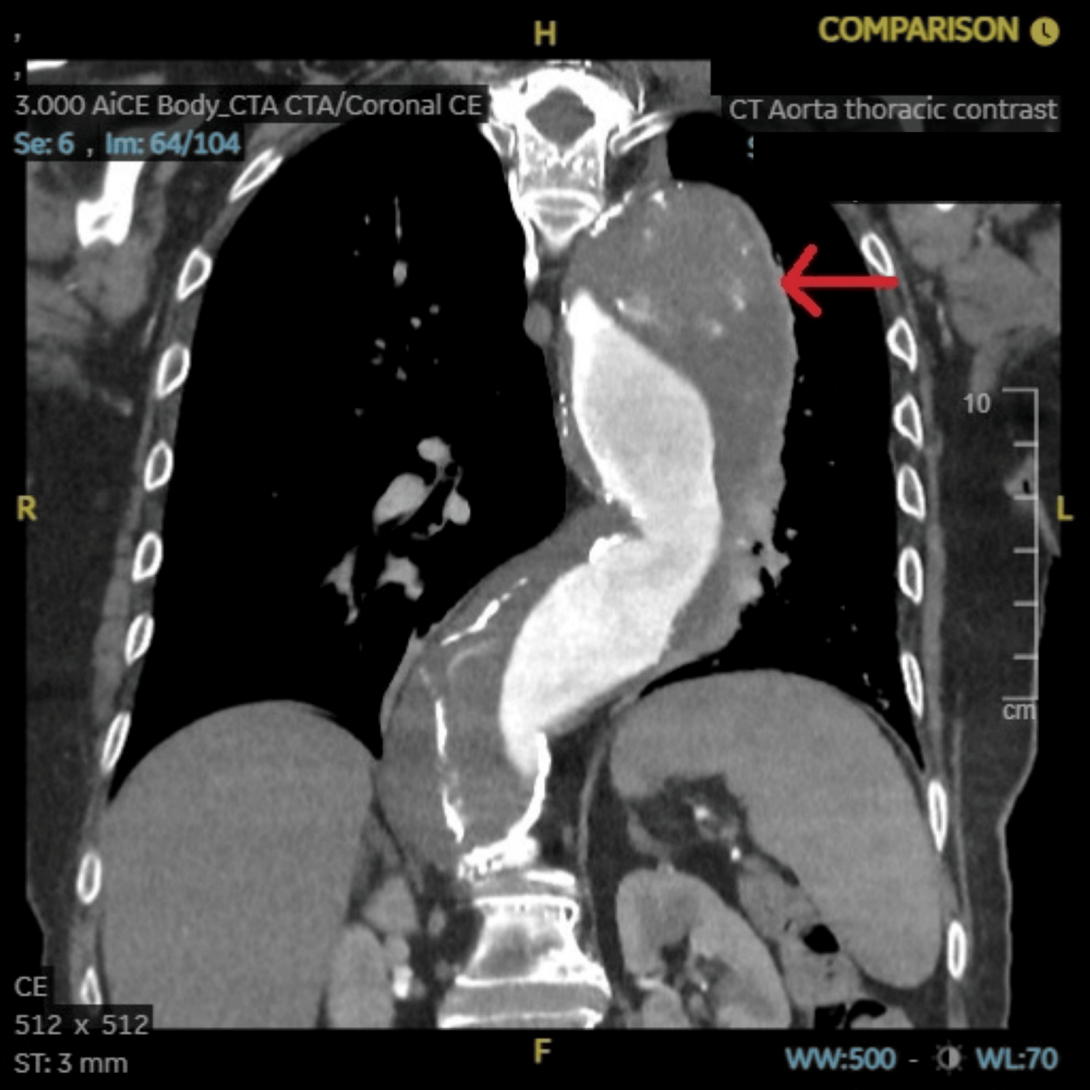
CT aortogram with contrast axial view showing a 78 x 78 mm large ruptured aneurysm of the descending aorta as highlighted by the red arrow.
